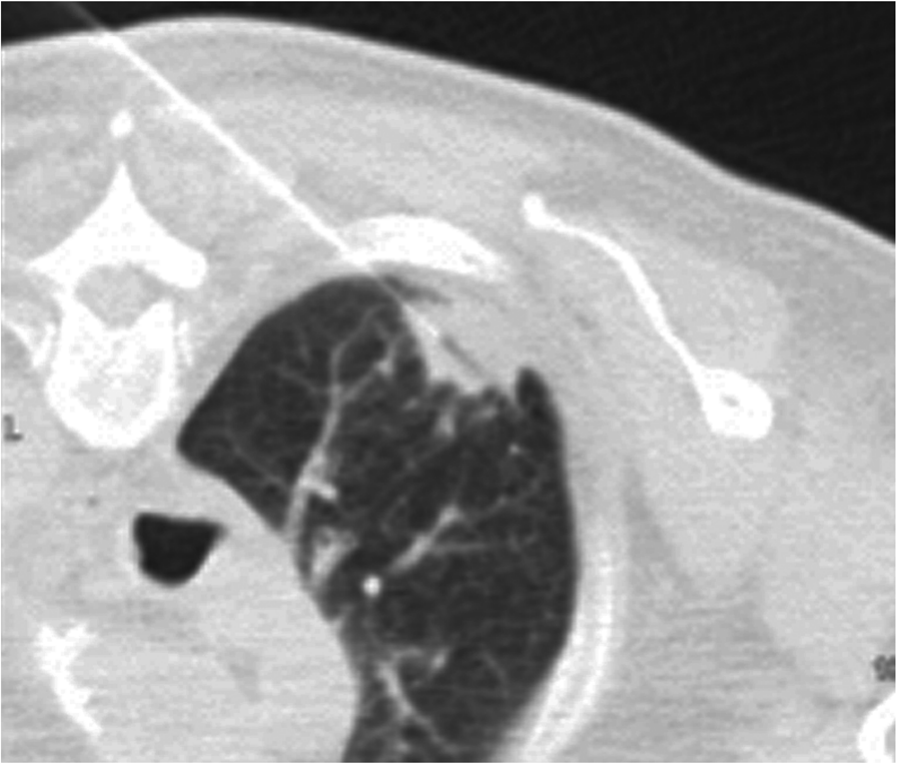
Computed tomography-guided fine-needle biopsy of the tumor in the upper lobe of the right lung
